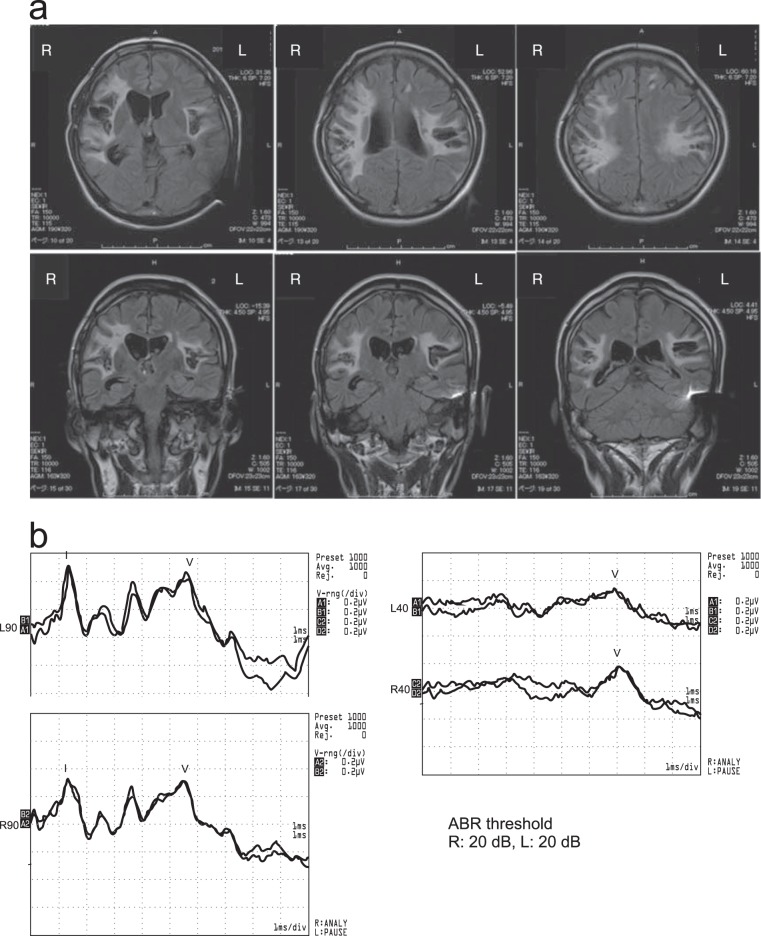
Details for patient exhibiting complete bilateral cortical deafness. Panel (a) shows an anatomical MRI of the patient showing bilateral lesions to auditory cortices, radiations, and postcentral gyruses. Panel (b) shows an intact auditory brainstem response (ABR) with thresholds of 20 dB in both ears. Additional details are provided in the Methods section. See Kaga *et al*.^21^ for original images and a more detailed patient description and testing protocols. Reproduced with permission.
